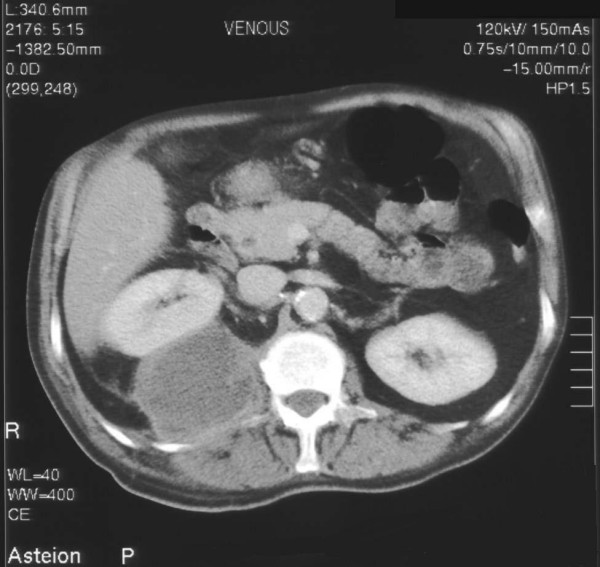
Computed tomogram of abdomen showing right psoas abscess abutting the right kidney.
